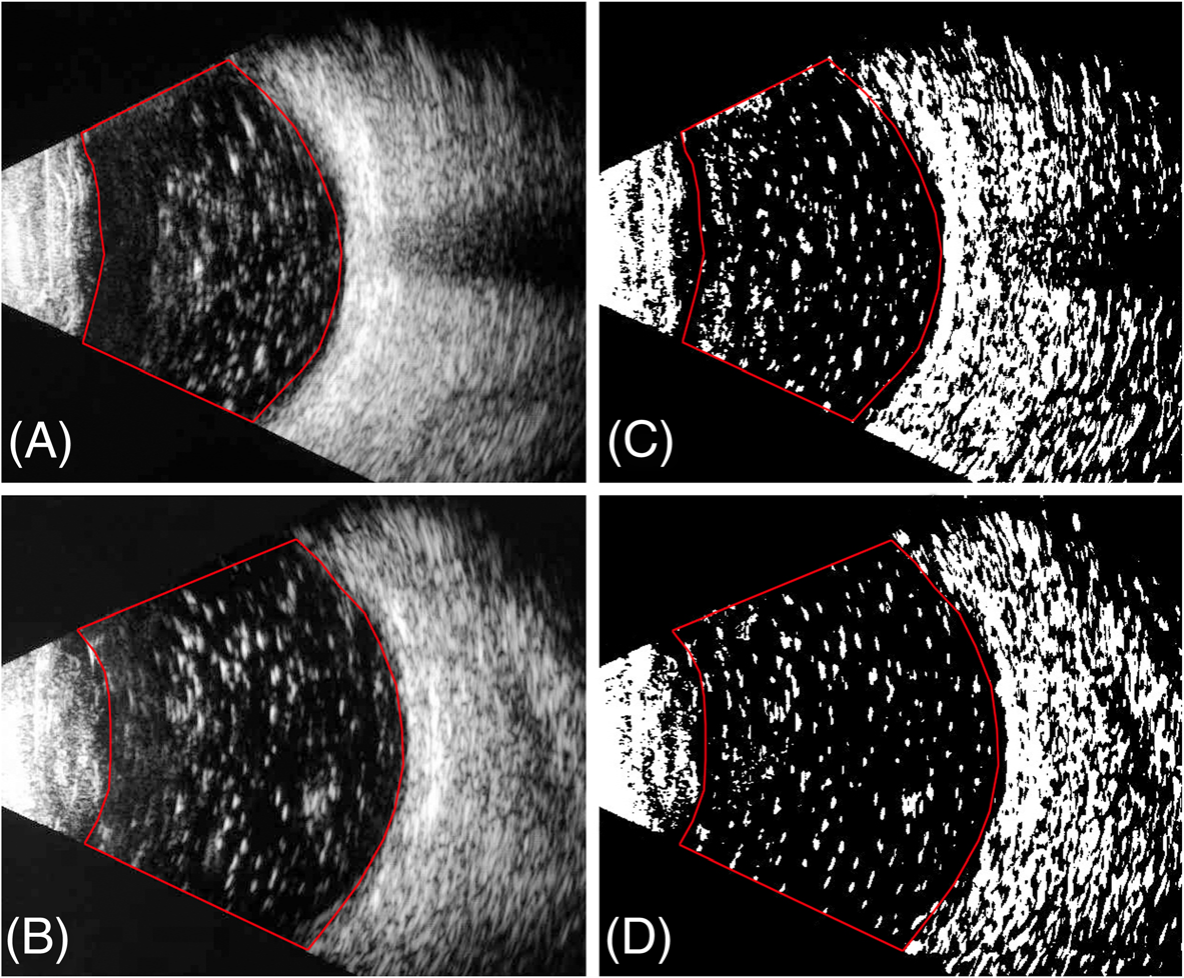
Postoperative silicone oil residuals. Ultrasonic image of Silicone oil residuals were magnified due to Rayleigh scattering. Representative images of patients who underwent silicone oil removal using transpupillary approach (a) and 23-gauge vitrectomy approach (b) were shown. Images were converted using a binarization method to quantify silicone oil remnants (c and d). The selected areas were analyzed and the results were presented as percentages
